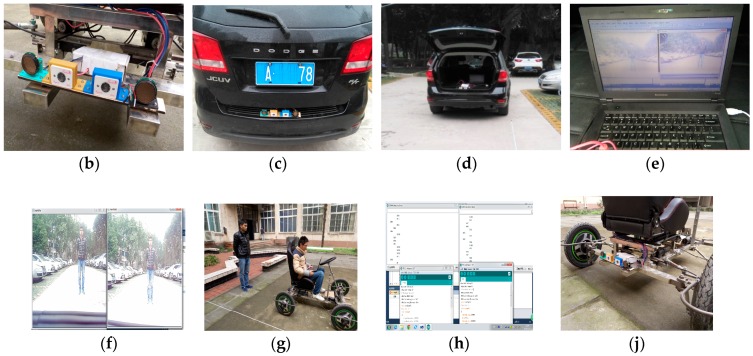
Experimental setup. (a) Experimental prototype; (b) multi-sensor in proposed system; (c) experimental vehicle; (d) test preparation; (e) test preparation; (f) binocular camera images; (g) obstacle detection using ultrasonic range finders; (h) tests using a homemade vehicle; (i) tests using a homemade vehicle.
